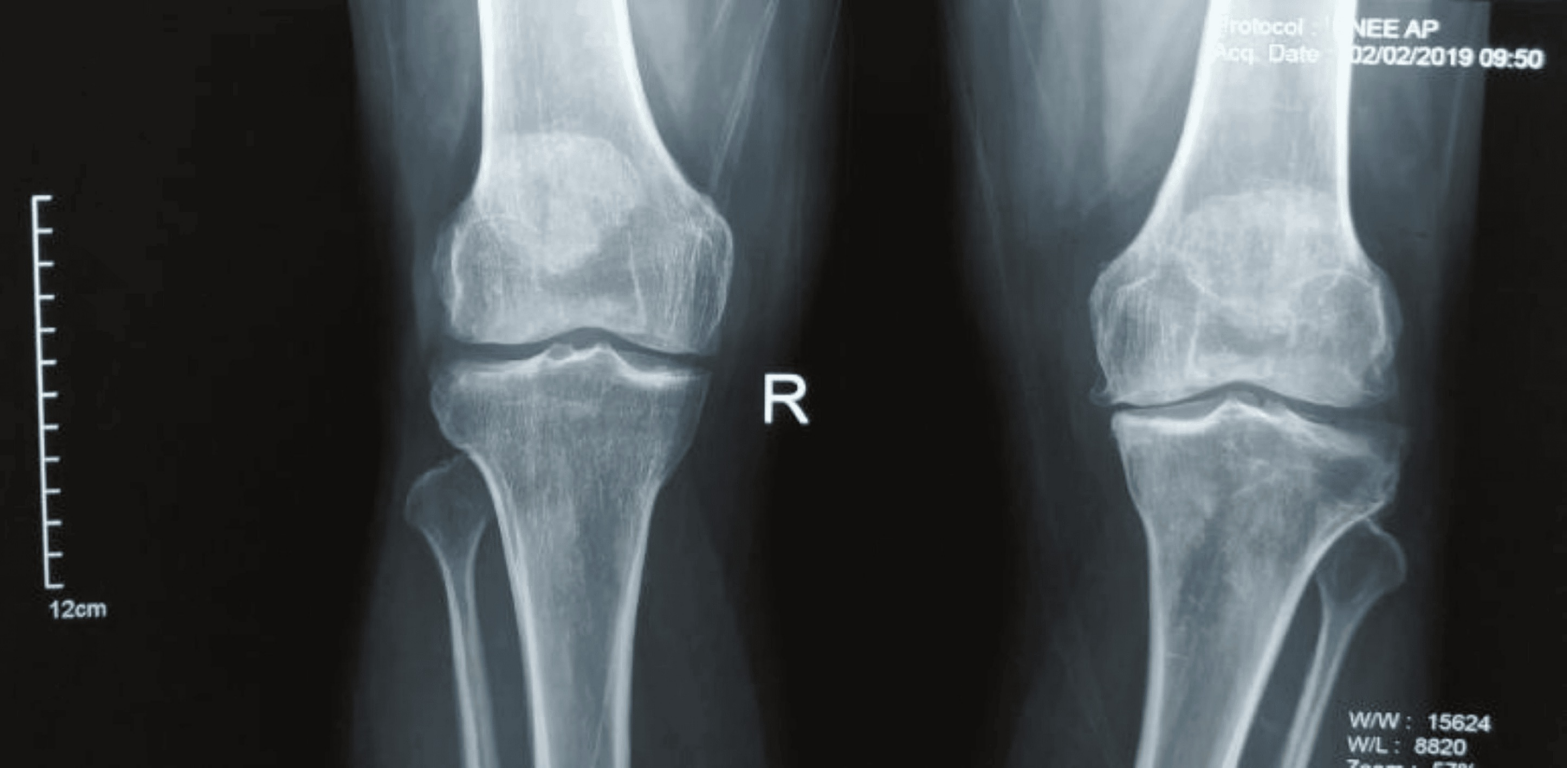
Preoperative radiograph of the patient with osteoarthritis of the right knee
